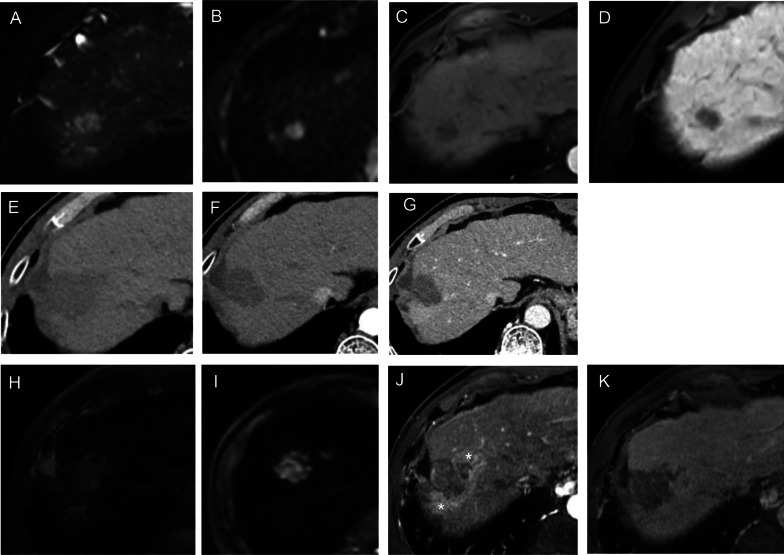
Images in a 56-year-old man with chronic hepatitis B virus infection. A–D Preoperative gadoxetate disodium–enhanced axial MRI scans show a 2.0-cm mass (NHHN) in segment VII, which shows moderate T2 hyperintensity (A), marked hyperintensity on DWI (b = 800 s/mm^2^) (B), without obvious enhancement on arterial phase image (C), marked hepatobiliary phase hypointensity (D). E–G Enhanced abdominal CT images of the patient 50 days after radiofrequency ablation treatment of tumor. A low-density ablation area was shown (E), and there was not any enhancement tissue in or along the margin of the treated lesion (F, G). Diagnosis was agreed upon by the 2 readers (LR-TR nonviable). H–K Gadoxetate disodium–enhanced axial MRI scans 67 days after tumor ablation, which shows T2 hyperintensity (H), marked hyperintensity on DWI (b = 800 s/mm^2^) (I), irregular thickened enhanced tissue area was found along the margin of the treated lesion (* in J), and marked hepatobiliary phase hypointensity (K). The recurrence-free survival for this patient was 60 days
